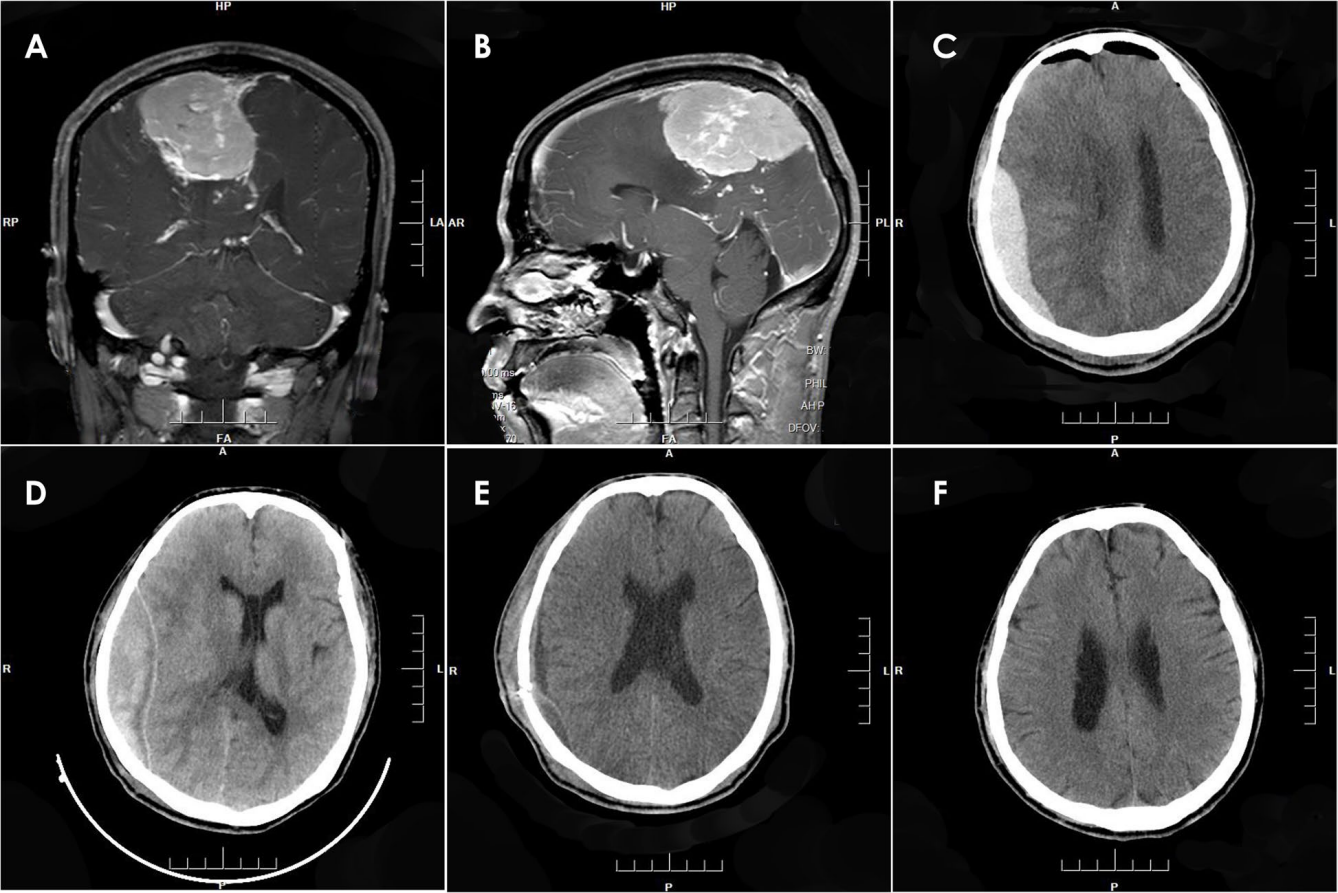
MRI with contrast enhancement revealing a giant parasagittal meningioma (A and B). CT scan showing a type 1 PEDH on the left frontal-parietal lobe (C). CT scan demonstrating the liquefaction of the EDH 2 weeks later (D). After the EDH evacuation via burr hole and drainage procedure, a CT scan was obtained (E). CT scan obtained 3 months later (F)
